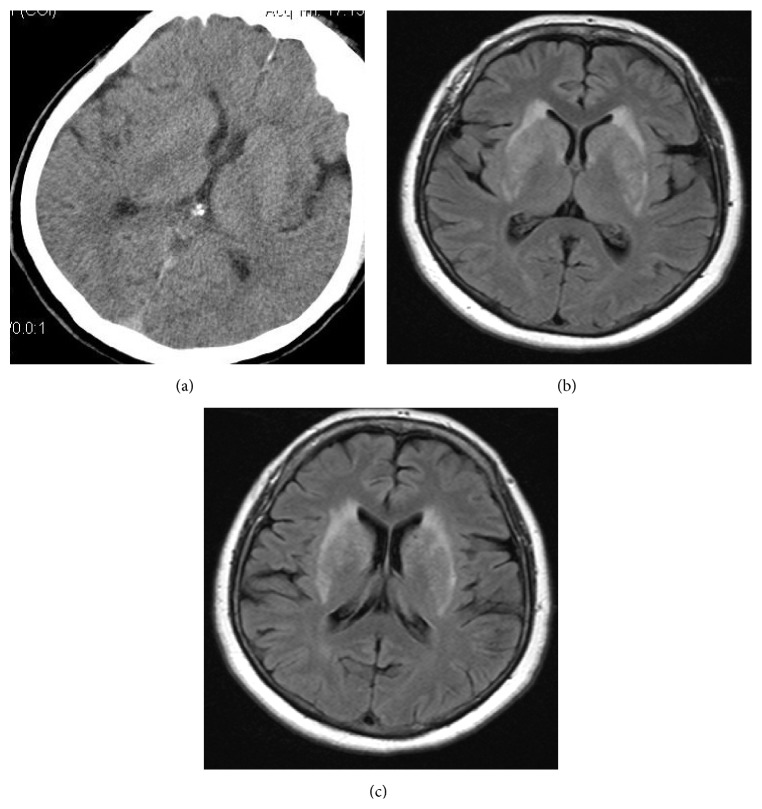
Noncontrast CT scan is normal (a) and FLAIR (fluid-attenuated inversion recovery) images demonstrate increased T2 signal activity at bilateral lentiform and caudate nucleus (b-c).
